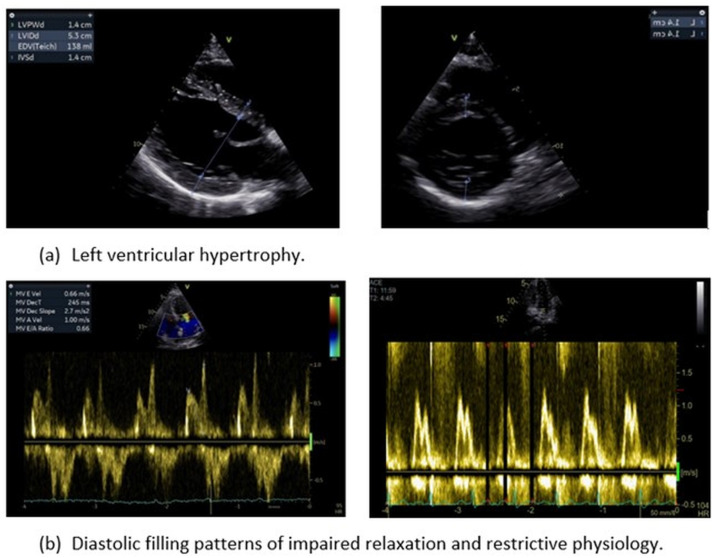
Classical echocardiographic indices of hypertensive heart disease.
